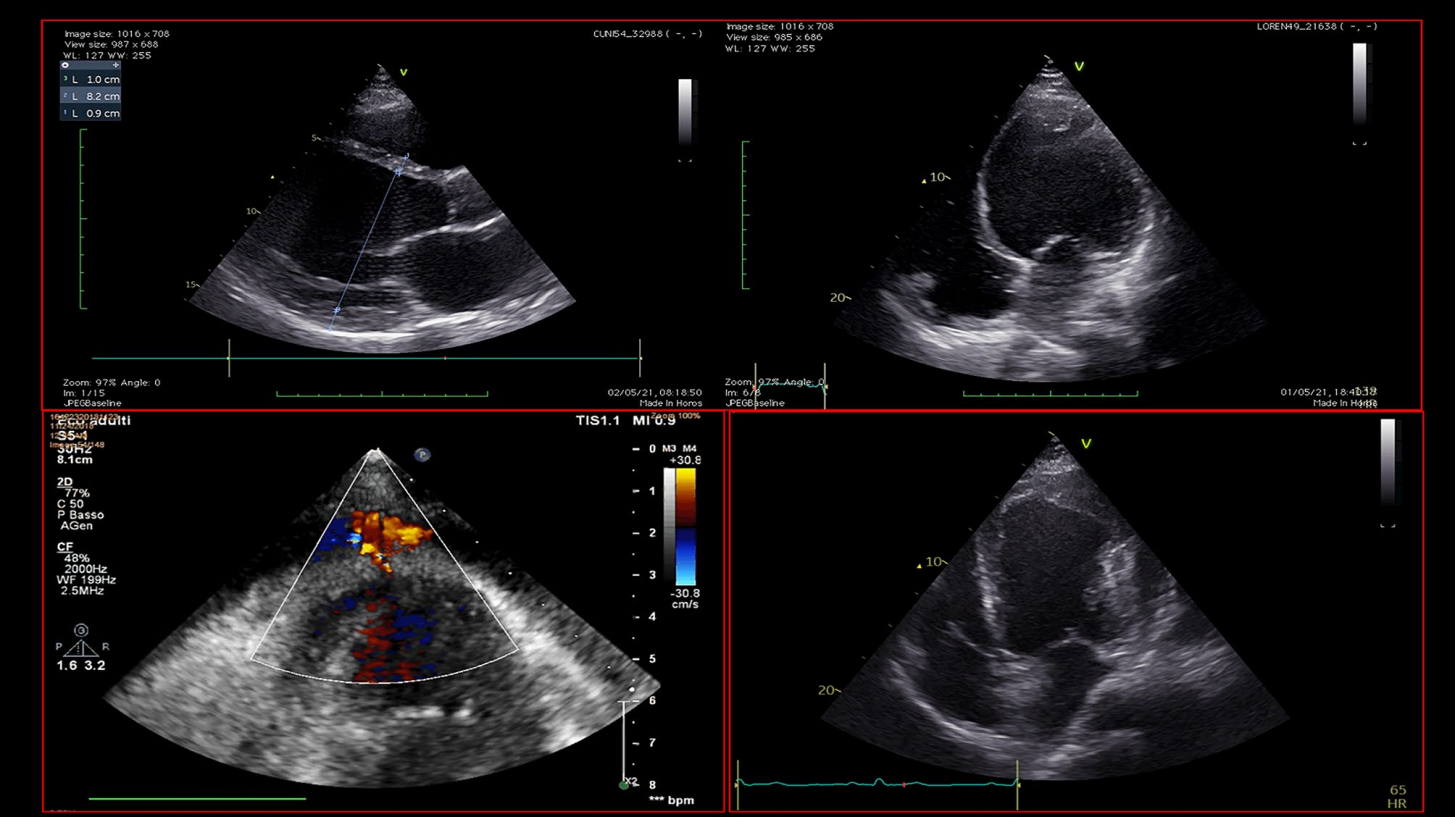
Echocardiographic assessment of patients admitted for cardiogenic shock. This picture shows different echocardiographic scenarios that can be found in patients admitted to intensive cardiac care unit for cardiogenic shock. The two images at the top show a severely dilated and impaired left ventricle with decreased wall thickness compatible with dilated cardiomyopathy, in presence of left ventricular thrombosis. The picture at the bottom left shows a left ventricular pseudoaneurism in a patient with a recent ST-elevation myocardial infarction, with flow passage demonstrated by Color Doppler. The picture at the bottom right shows a finding suspicious for left ventricular aneurism or pseudoaneurism, in presence of extensive thrombosis
